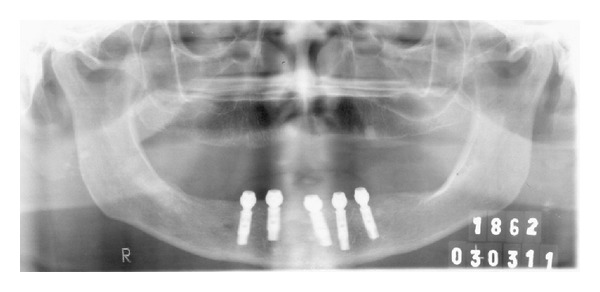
Panoramic radiography after implants placement.
